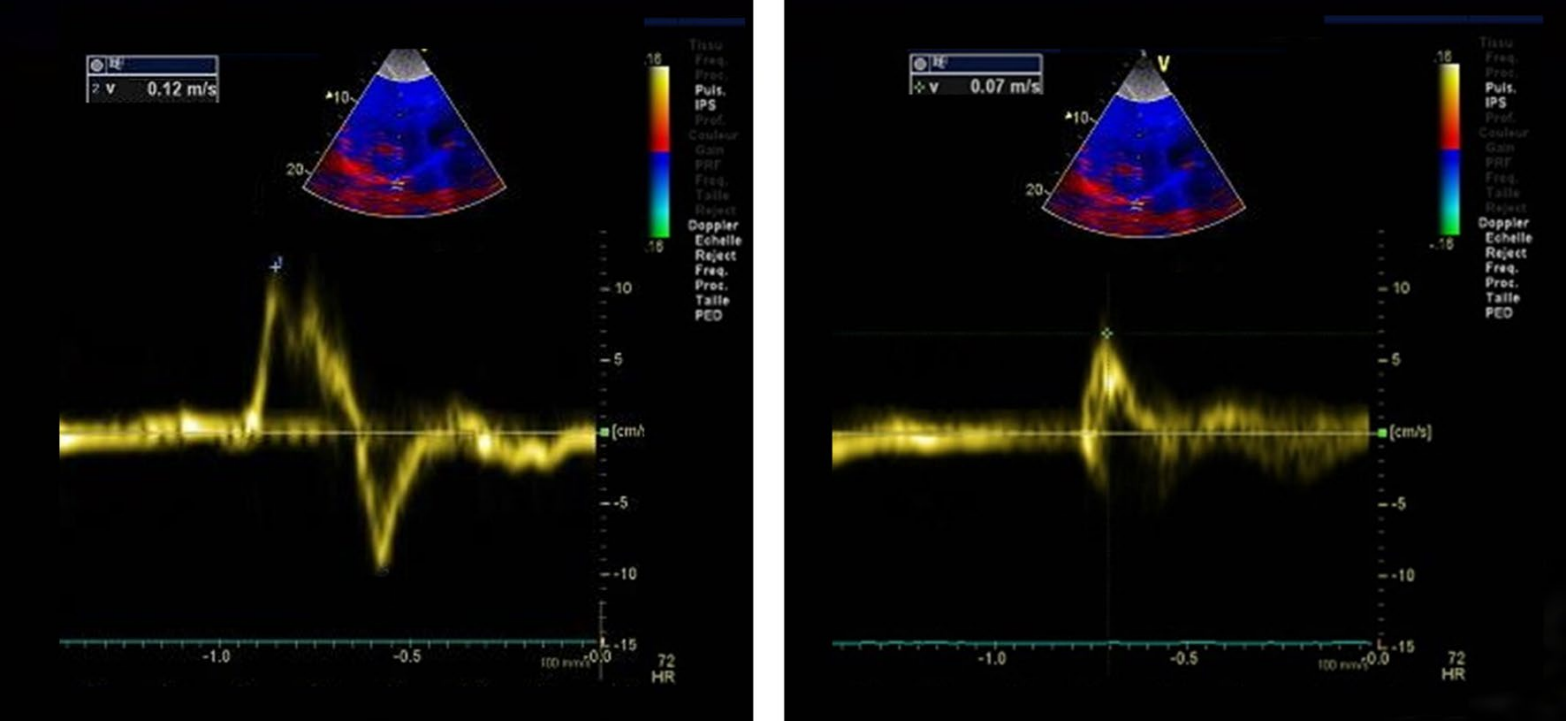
Representative tracings of peak velocity of the right hemidiaphragm during a sniff maneuver using tissue Doppler imaging in a healthy volunteer (*left panel*) and in a patient with Duchenne muscular dystrophy (*right panel).* The peak velocity in the healthy subject (12 cm/s) was nearly as twice as fast as the peak velocity in the patient Duchenne muscular dystrophy (7 cm/s). Reproduced under Open Access Creative Commons License: Fayssoil et al. PLoS One 2019;14(4):e0214288
